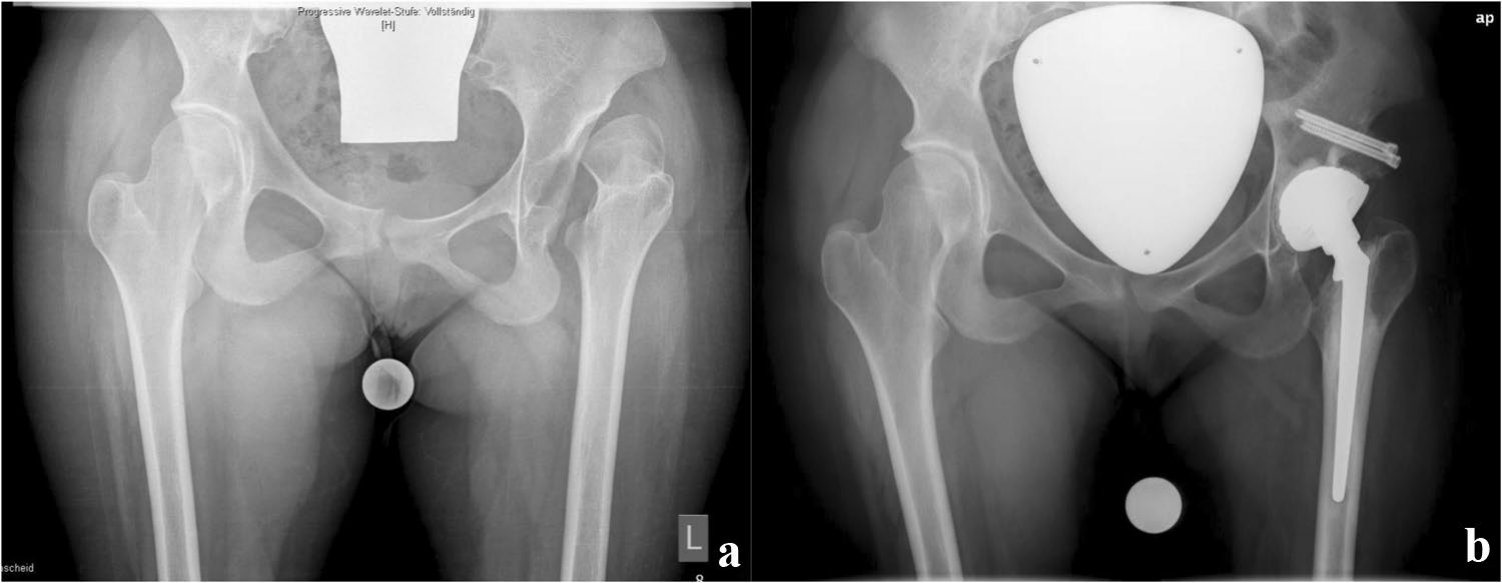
a Preoperative radiograph showing chronic hip dislocation on the left with osteoarthritic changes. b Postoperative radiograph after THA and concomitant acetabular augmentation with the femoral head
